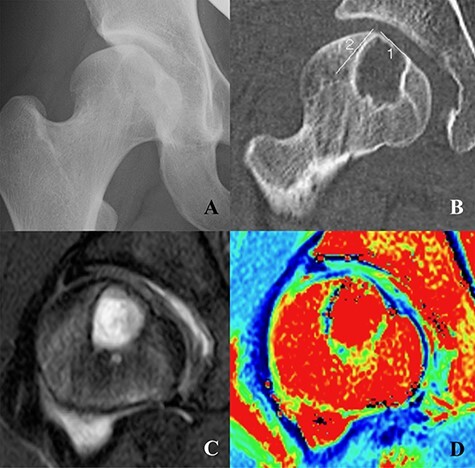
(A) A round osteolytic lesion suggesting a large bone cyst at the superior portion of the right femoral head. (B) Preoperative coronal CT showing a round bone cyst at the femoral head. (C) Coronal MRI showing a high-intensity round lesion at the femoral head. (D) T2 mapping showing cartilage of the femoral head. There was no evidence of cartilage damage.
